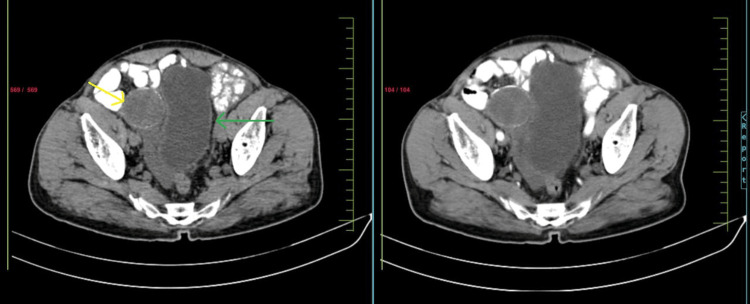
CECT abdomen-plain and arterial phase representing mucocele of the appendix (axial cut section) The yellow arrow shows mucocele and the green arrow indicates the bladder CECT: contrast-enhanced computed tomography
